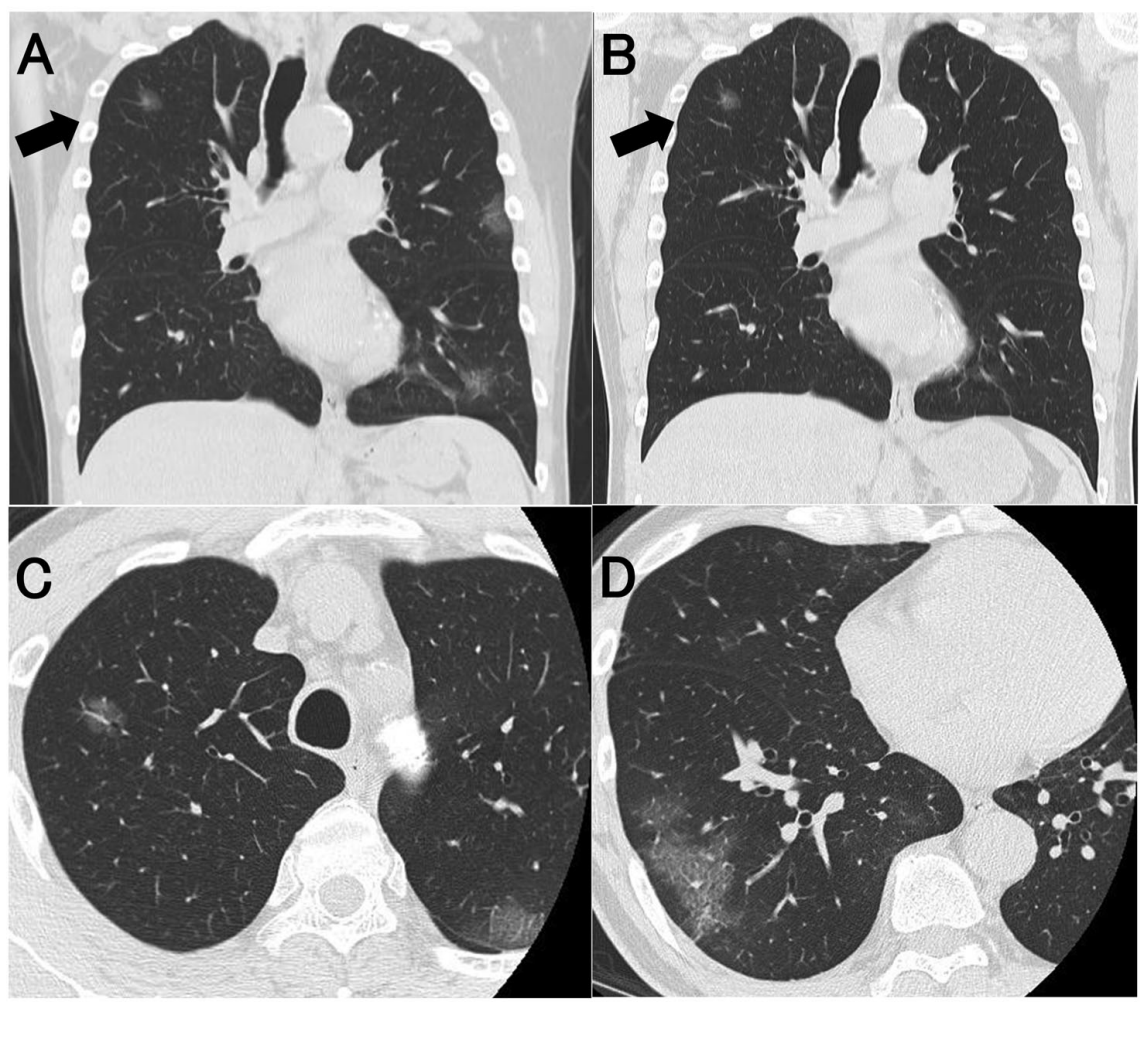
Coronavirus disease 2019 (COVID‐19) pneumonia and a primary lung adenocarcinoma. Chest computed tomography (CT) shows multiple patchy ground‐glass nodules, predominantly distributed in the peripheral lung parenchyma of the lower lobes in coronal section (A). Follow‐up CT three months after the first one shows that the ground‐glass nodule (GGN) (arrow) in the right upper lobe is still obvious (B). Thin‐slice CT of adenocarcinoma in situ (AIS) (C) and COVID‐19 pneumonia (D) in axial section. GGNs (D) are distributed in the periphery and subpleura, a typical finding in COVID‐19 pneumonia. In addition, peripheral GGNs (D) with superimposed intralobular reticulations resulting in a crazy‐paving pattern are shown [2]. A GGN (C), on the other hand, is lacking in these characteristic findings.
